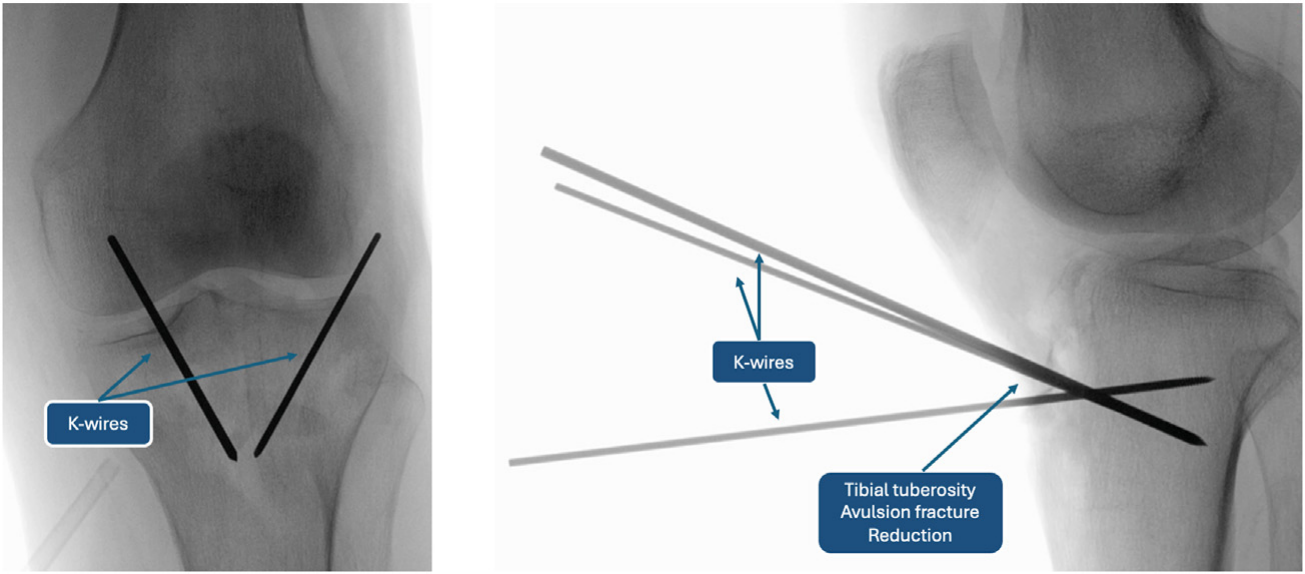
Temporary fixation of tibial tuberosity with K-wires: anteroposterior (left) and lateral (right) knee radiographs.
